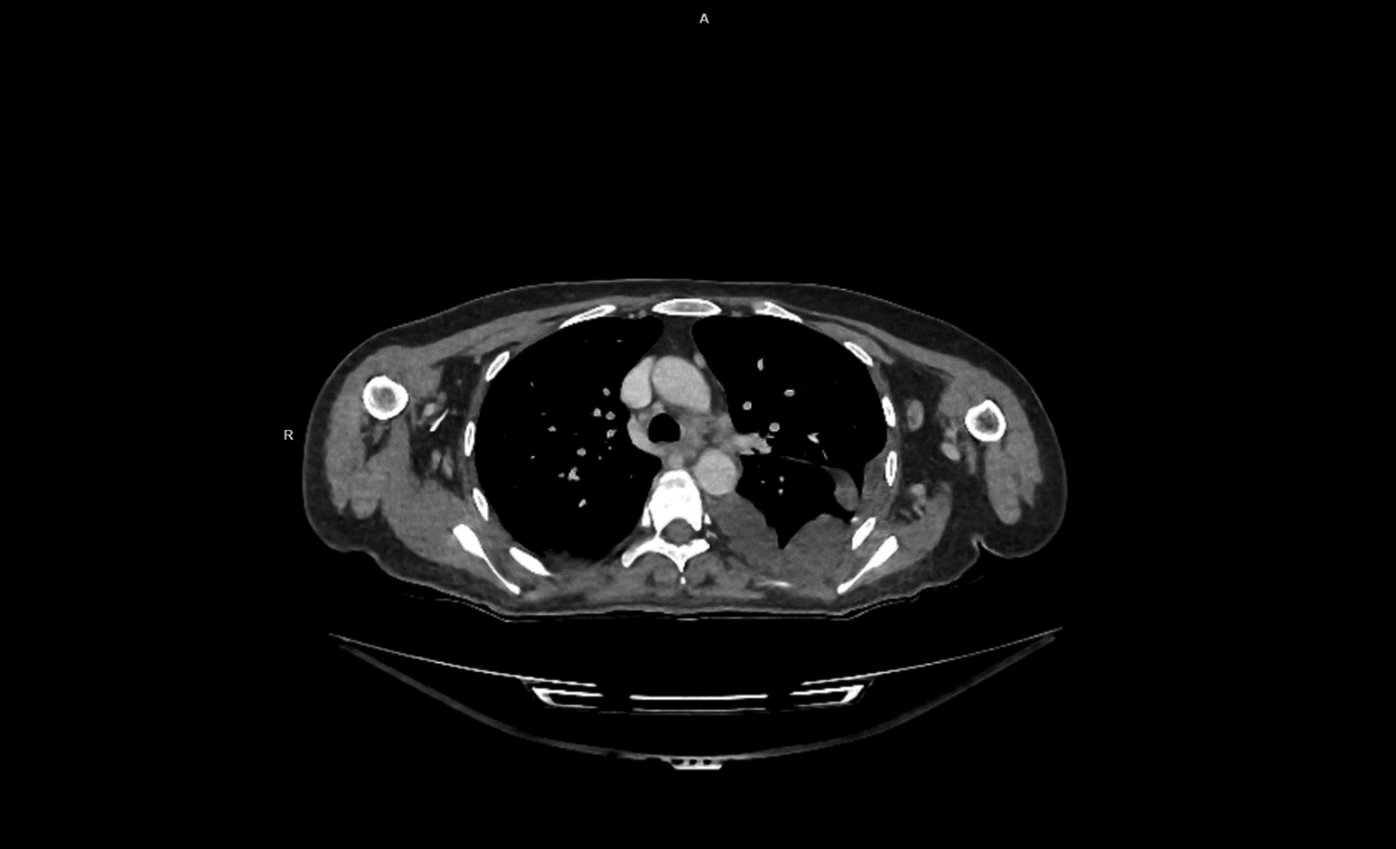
Thorax CT showing mediastinal lymphadenopathies.
